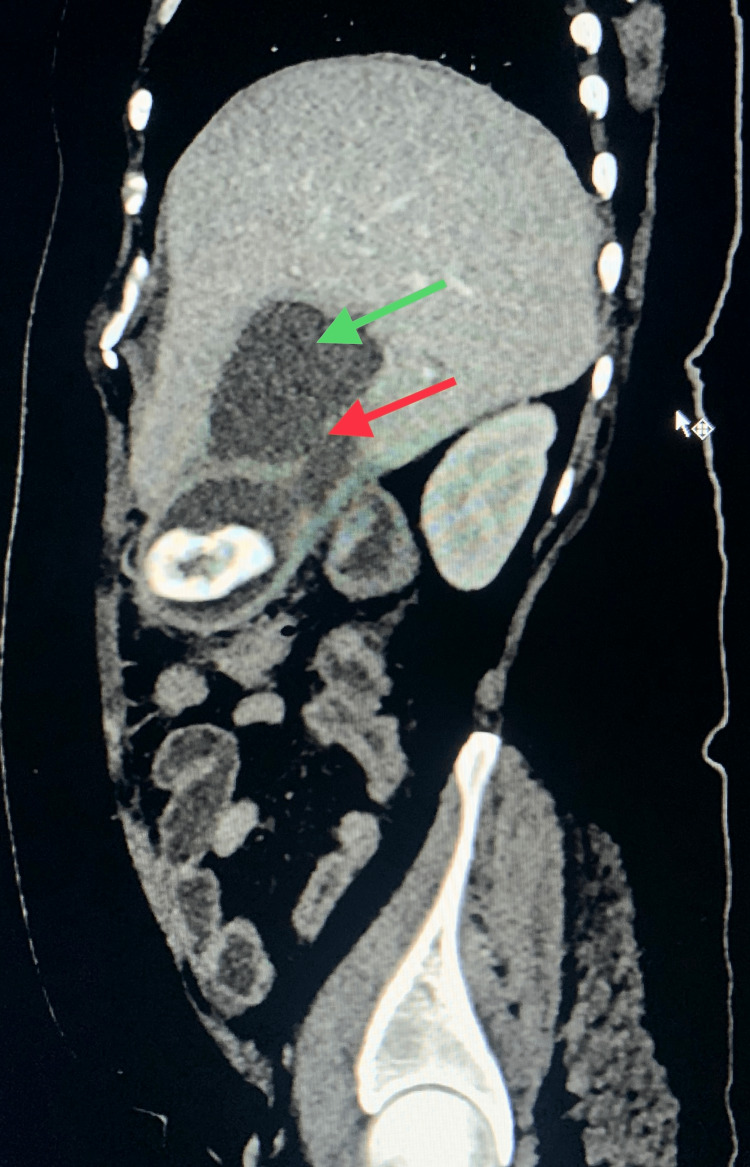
CT sagittal view showing a large gallstone with a defect in the GB wall (red arrow) and an adjacent hepatic segment IV abscess (green arrow) CT: computerized tomography, GB: gallbladder.
